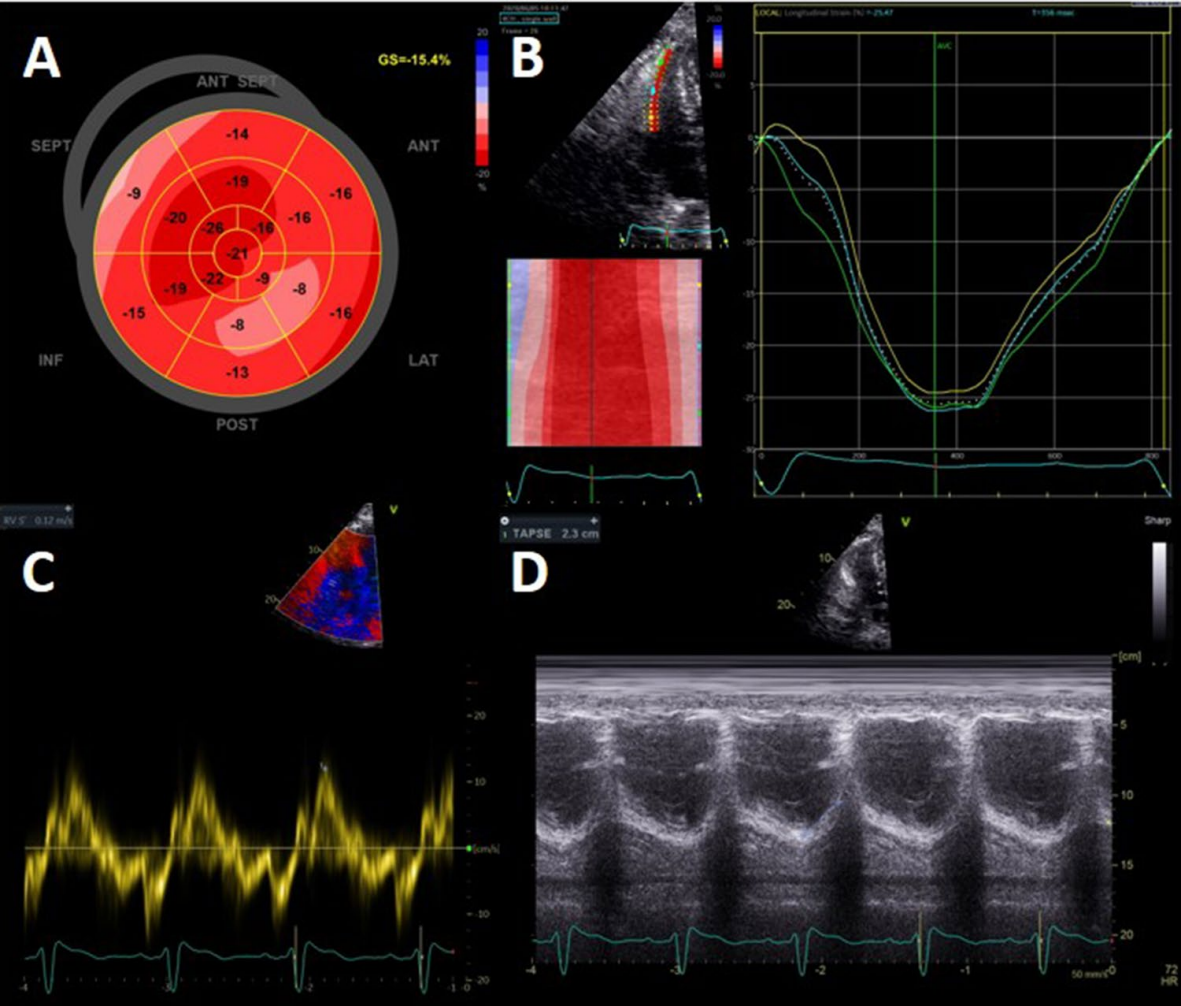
Case example of a COVID-19 patients with mild echocardiographic abnormalities. Example of a 75 year old patient with a history of diabetes and prostate cancer, he was admitted to the general ward for COVID-19 for 6 days in the first wave. During admission there were signs of cardiac injury (i.e., elevated troponin). At the out-patient clinical the patient has residual NYHA II dyspnoea symptoms. Echocardiography showed a mildly reduced left ventricular function (LVEF 54%, GLS − 15%). RV function was normal (TAPSE 23 mm, RV Sʹ 12 cm/sec, RV strain − 25%)
